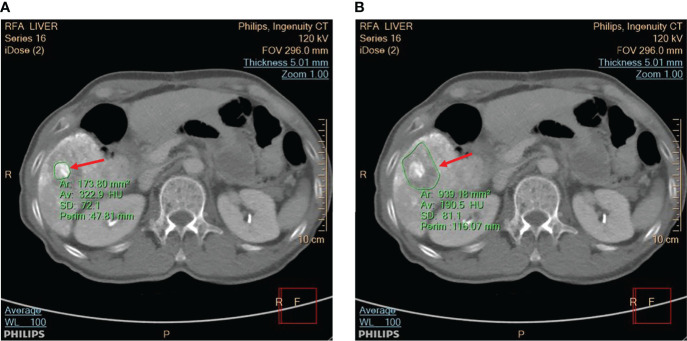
The CT scan was utilized to view (A) the tumor and (B) the ablation zone, as indicated by red arrows. The application IntelliSpace Portal (Philips) was utilized to measure the tumor size and ablation zone size, as shown in the CT images segmented by green contour lines.
